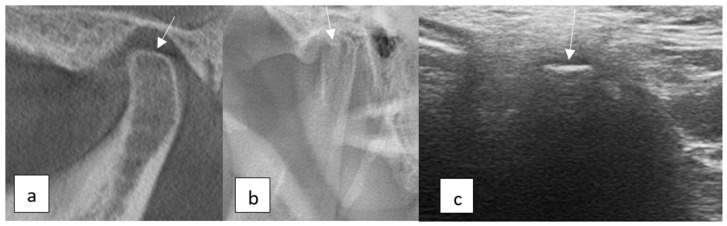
TMJ flattening: (a) CBCT image showing flattening in the superior surface of the condyle (sagittal view), (b) panoramic radiography image, and (c) USG image.
